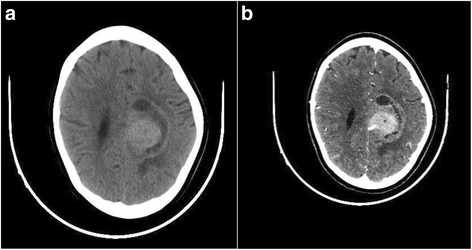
Computed tomography scan revealing a voluminous mass in the perilateral ventricle with similar attenuation to that of cortical gray matter (a), following administration of a contrast product, the mass showed marked enhancement (b)
